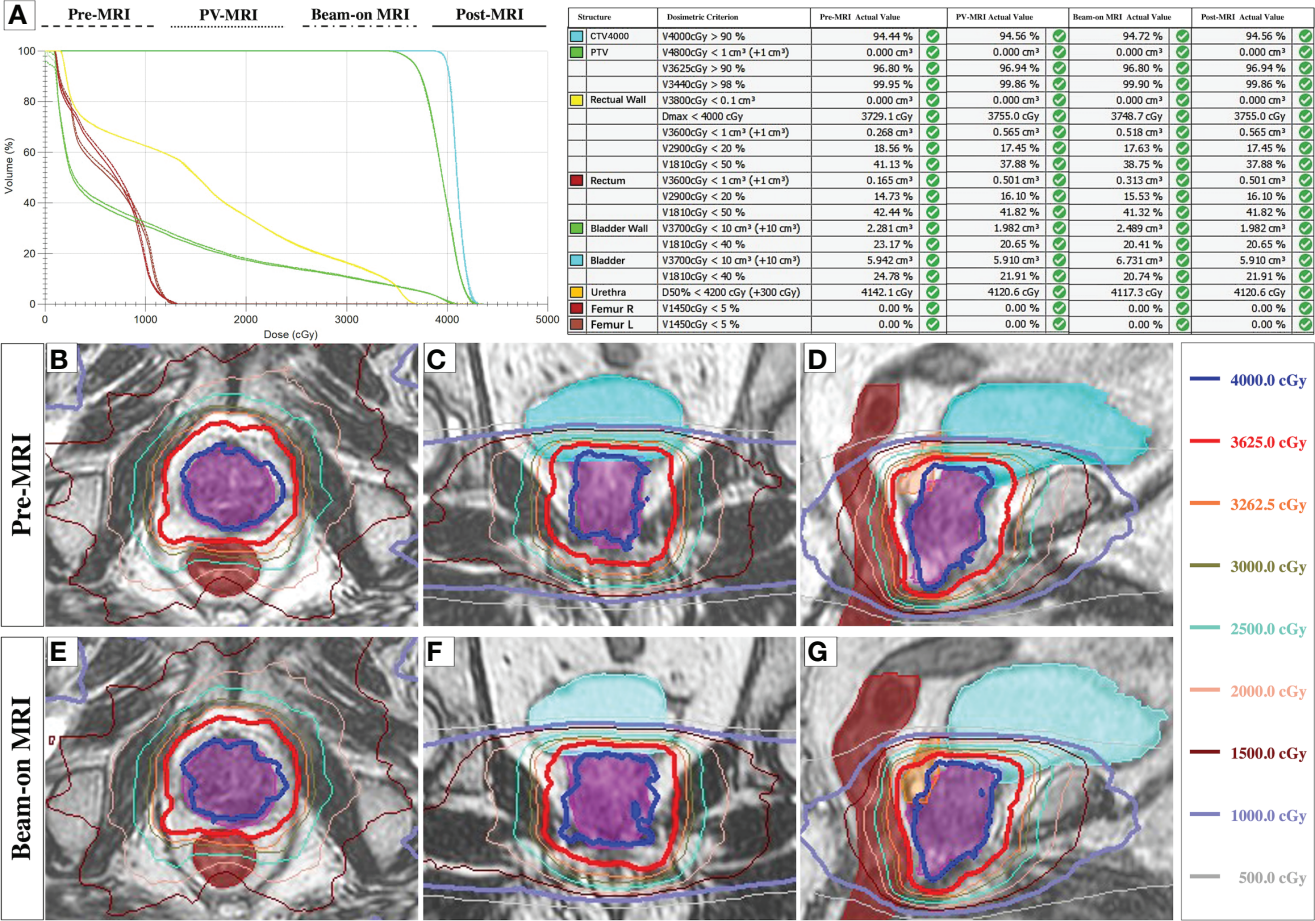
The dose distributions on each MRI scans. (A): The representative DVH plot with four plans and the dose metrics on each MRI scans after re-planning in one fraction. (B–G): The representative dose distributions of three planes of ATS plan on Pre-MR (B–D) and Beam-on MR scan (E–G).
